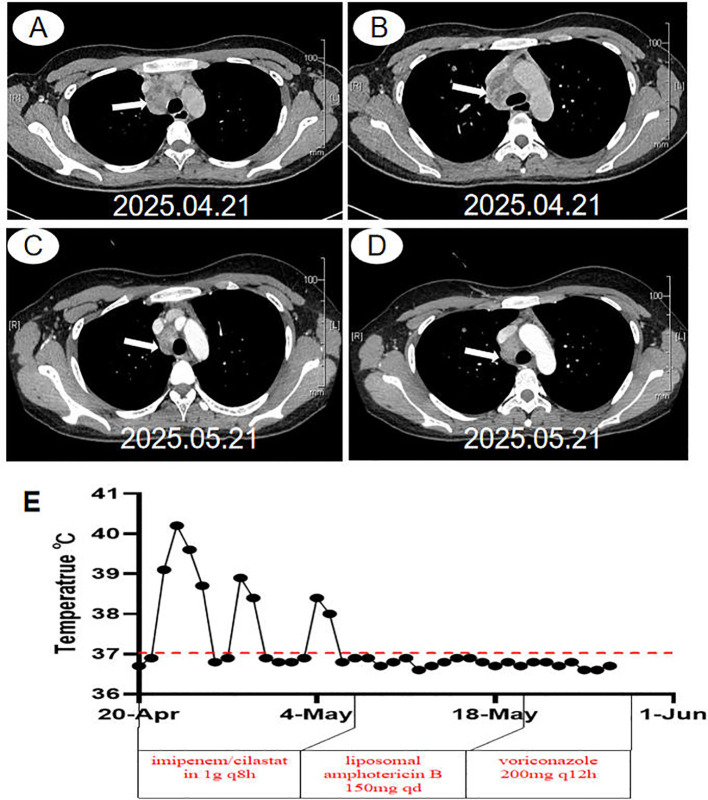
Chest computed tomography images and the changes in temperature and drug use of the patient at the first visit. (A, B) Initial contrast-enhanced chest CT revealing a 4.0-cm × 3.8-cm mass in the upper–middle mediastinum (*white arrow*). The mass demonstrates heterogeneous internal density and heterogeneous enhancement, with associated compression of the superior vena cava. (C, D) Follow-up chest CT performed on the 14th day of treatment showing a marked reduction in the size of the mediastinal lesion, which measures approximately 2.8 cm × 2.0 cm (*white arrow*). (E) Changes in temperature and drug use of the patient at the first visit.
